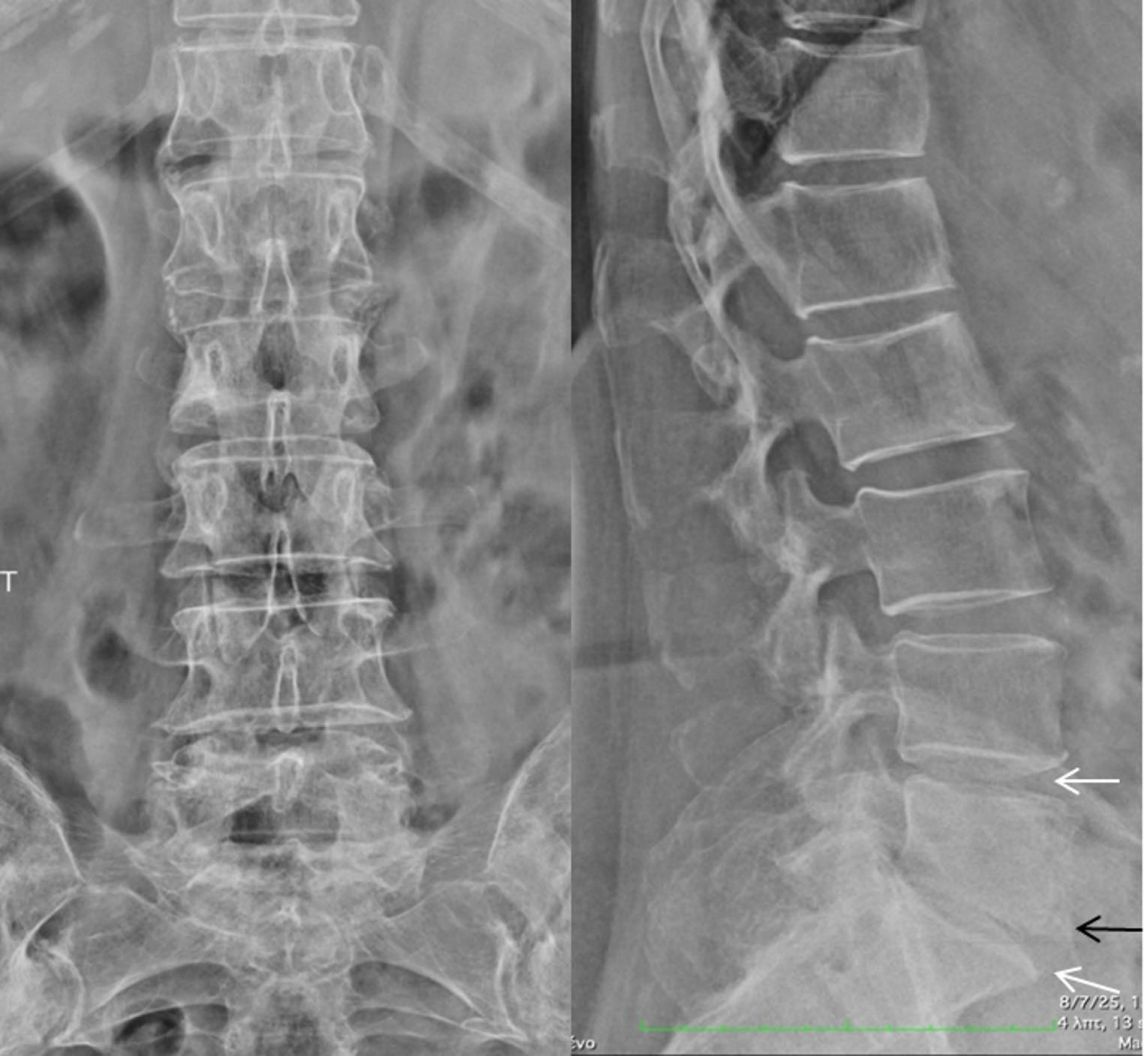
Lumbar spine x-rays, anteroposterior (a) and lateral (b) views. Note the spinal disc space narrowing between the L4-L5 and L5-S1 vertebrae (white arrows) and the presence of osteophytes at the L5-SI level (black arrow).
